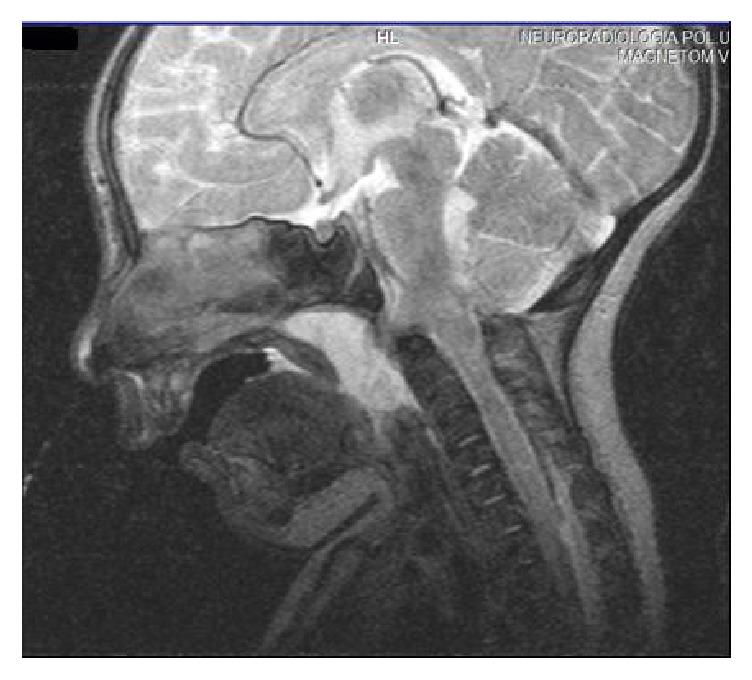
Sagittal T2W MRI of the skull shows a basal occipital meningoencephalocele with protrusion toward the naso- and oropharynx. The content of this malformation was primarily fluid with a small quantity of impacted bulb brain parenchyma.
